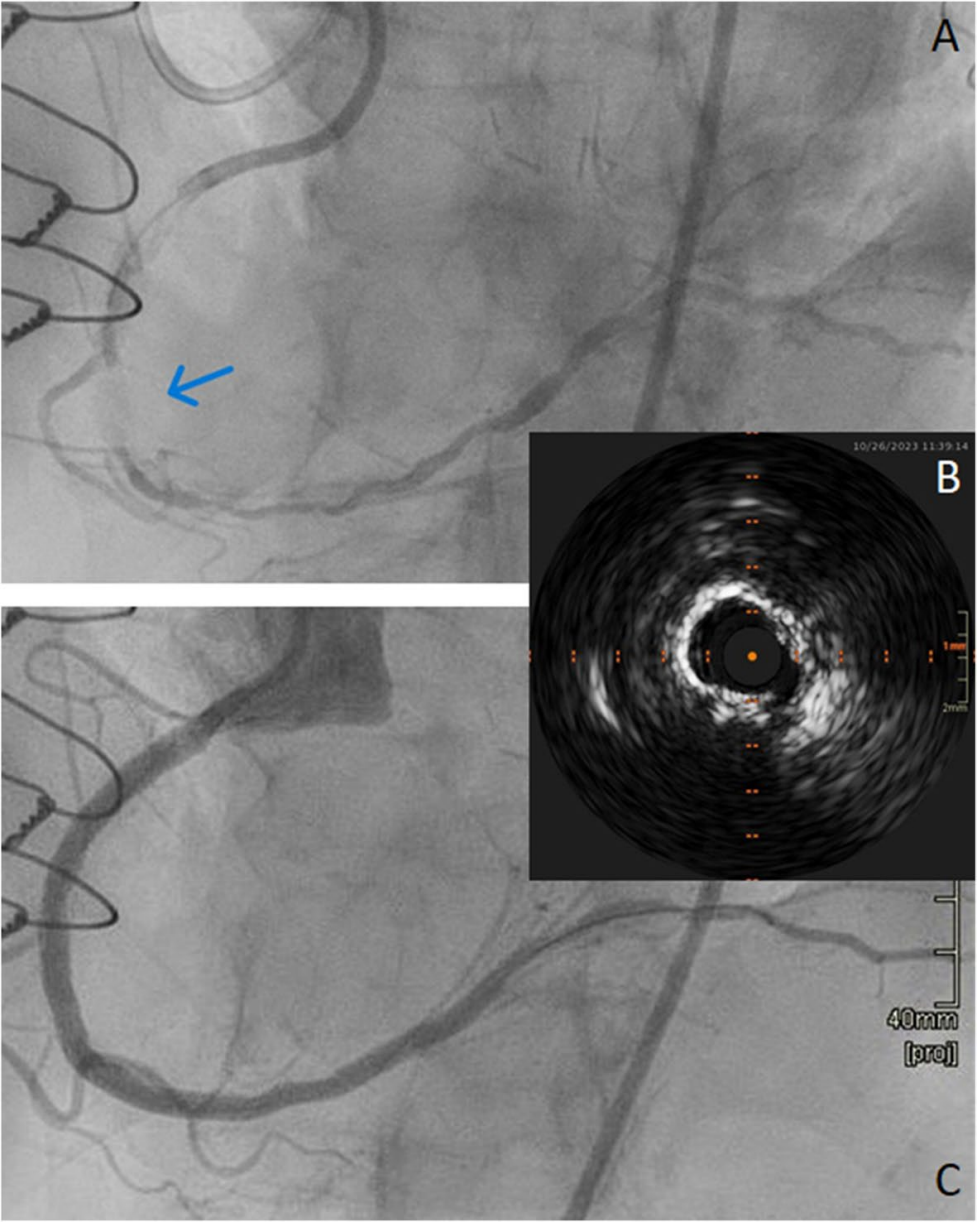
Coronary angiography and intravascular ultrasound image of the right coronary artery. A Right coronary artery chronic total occlusion (blue arrow) before treatment with Shockwave Intravascular Lithotripsy and percutaneous coronary intervention. B Intravascular imaging of the right coronary artery showing circular calcification. C Final result after percutaneous coronary intervention of the right coronary artery
